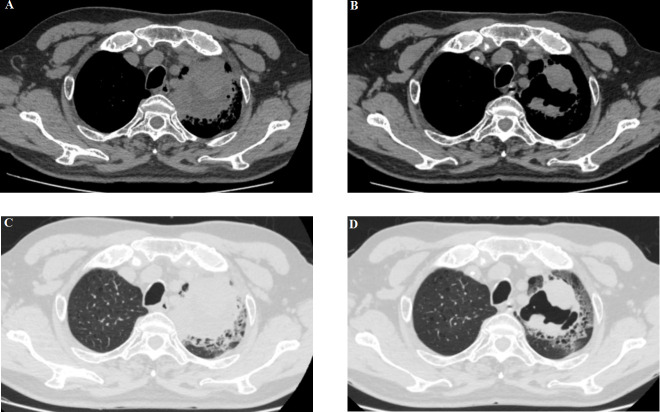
(A, C) A baseline computed tomography scan taken before treatment with ICI therapy, demonstrating the left lung mass. (B, D) A computed tomography scan taken at the time of BP development (6 weeks after pembrolizumab discontinuation), showing a partial response.
